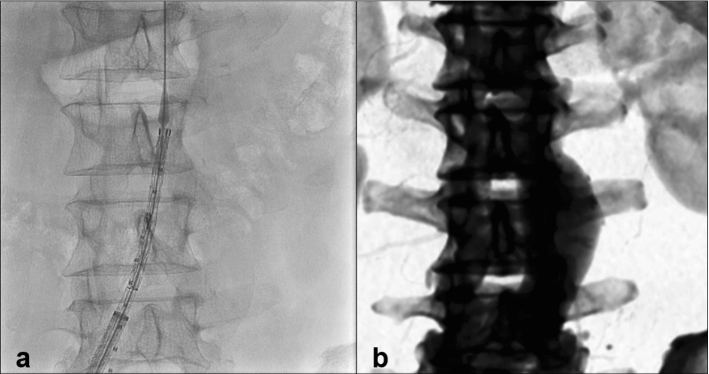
Imaging used for automated fusion: first fluoroscopy image of the initial DSA (a) and preoperative CTA converted to a digital X-ray projection (b)
